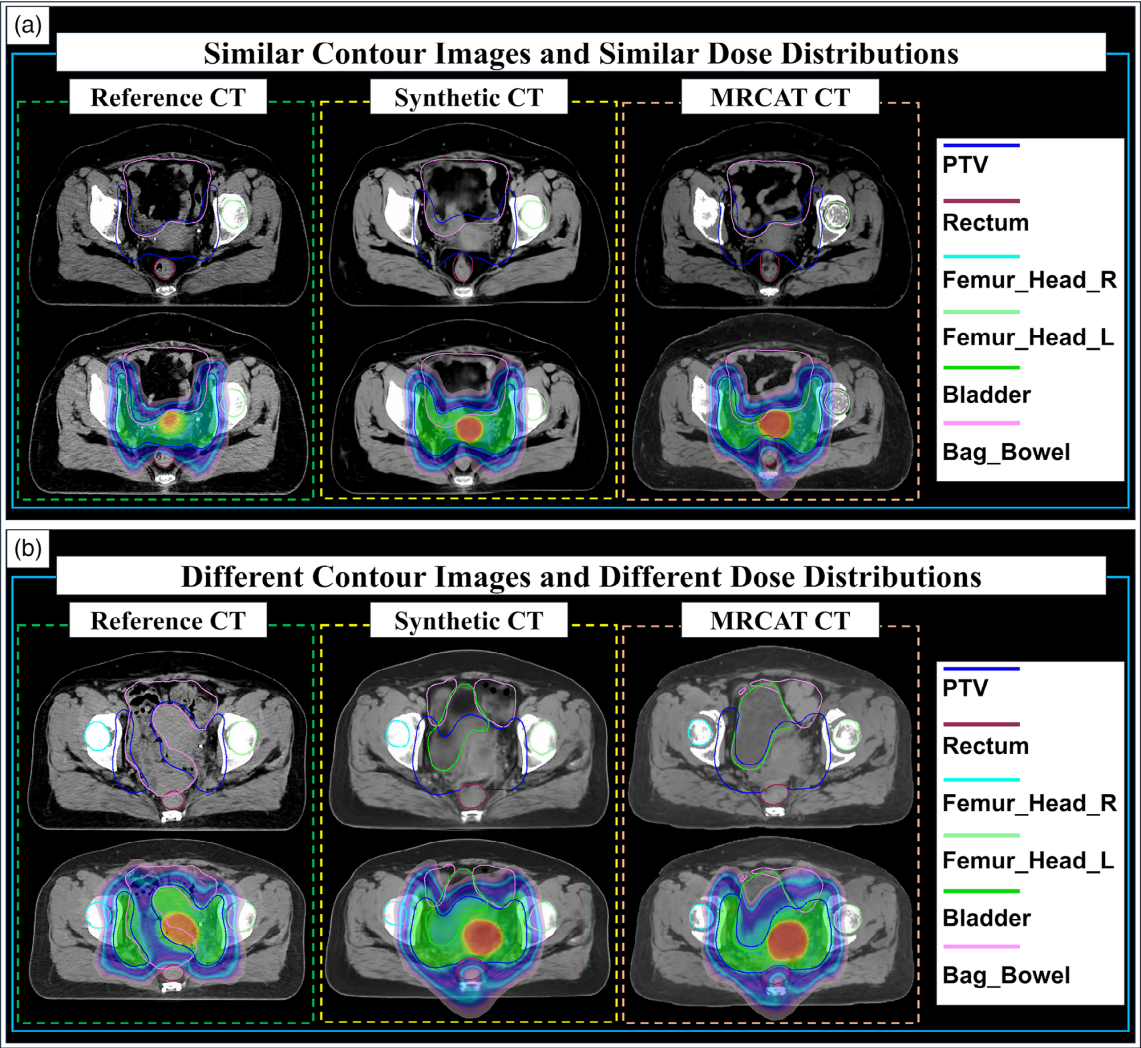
Comparison of contour images and dose distribution maps for the same treatment plan. (a) Similar contour images and dose distribution maps for the same computed tomography (CT) slice number for patient 3; (b) Different contour images and dose distribution maps for the same CT slice number for patient 1.
